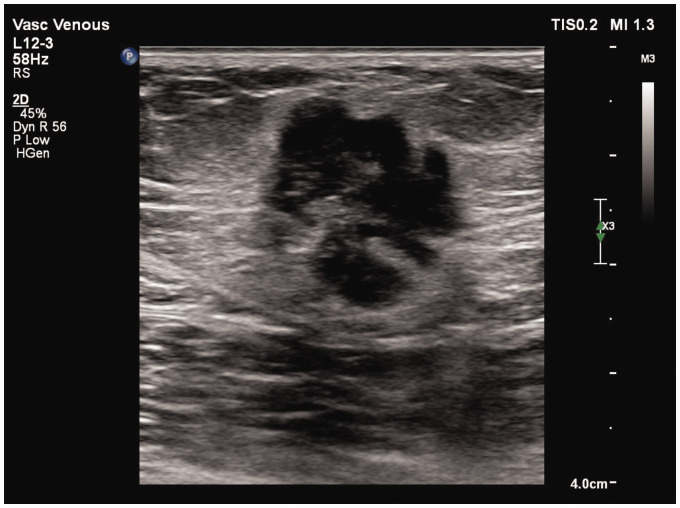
Ultrasound image demonstrating a subcutaneous heterogeneous hypoechoic collection, underlying the erythematous lesion of the patient’s thigh.
